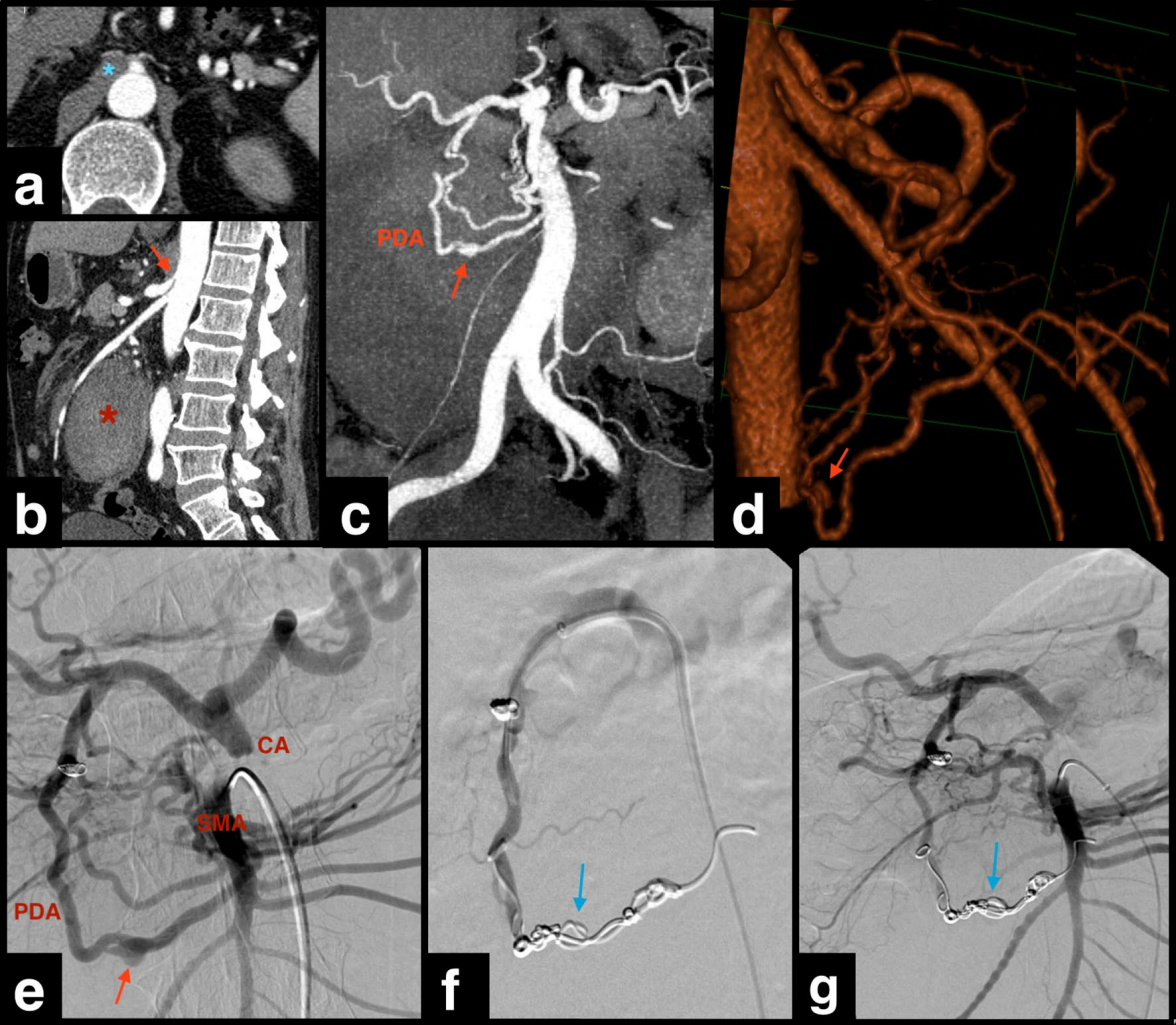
Ruptured aneurysm of the anterior inferior pancreaticoduodenal artery (PDA) in a patient with celiac artery stenosis. Axial (a) and sagittal (b) CTA images show the thickened median arcuate ligament (* in a), celiac artery compression (arrow), and a blood collection (* in b) secondary to rupture of a PDA aneurysm (arrow in c, coronal MIP reconstruction, and d, 3D reconstruction). Selective superior mesenteric artery (SMA) angiogram (e) shows severe celiac artery (CA) stenosis and the PDA aneurysm (red arrow). After selective catheterization (f), both artery and aneurysm were embolized with microcoils (blue arrows). Post-embolization angiogram (g) shows no residual aneurysm opacification. A “string-of-beads” appearance was noted in the ileocolic artery, indicative of segmental arterial mediolysis or fibromuscular dysplasia (not confirmed), suggesting an exacerbating and not only causative role of MALC in aneurysm formation and rupture. CTA, computed tomography angiography; MIP, maximum intensity projection; SMA, superior mesenteric artery
